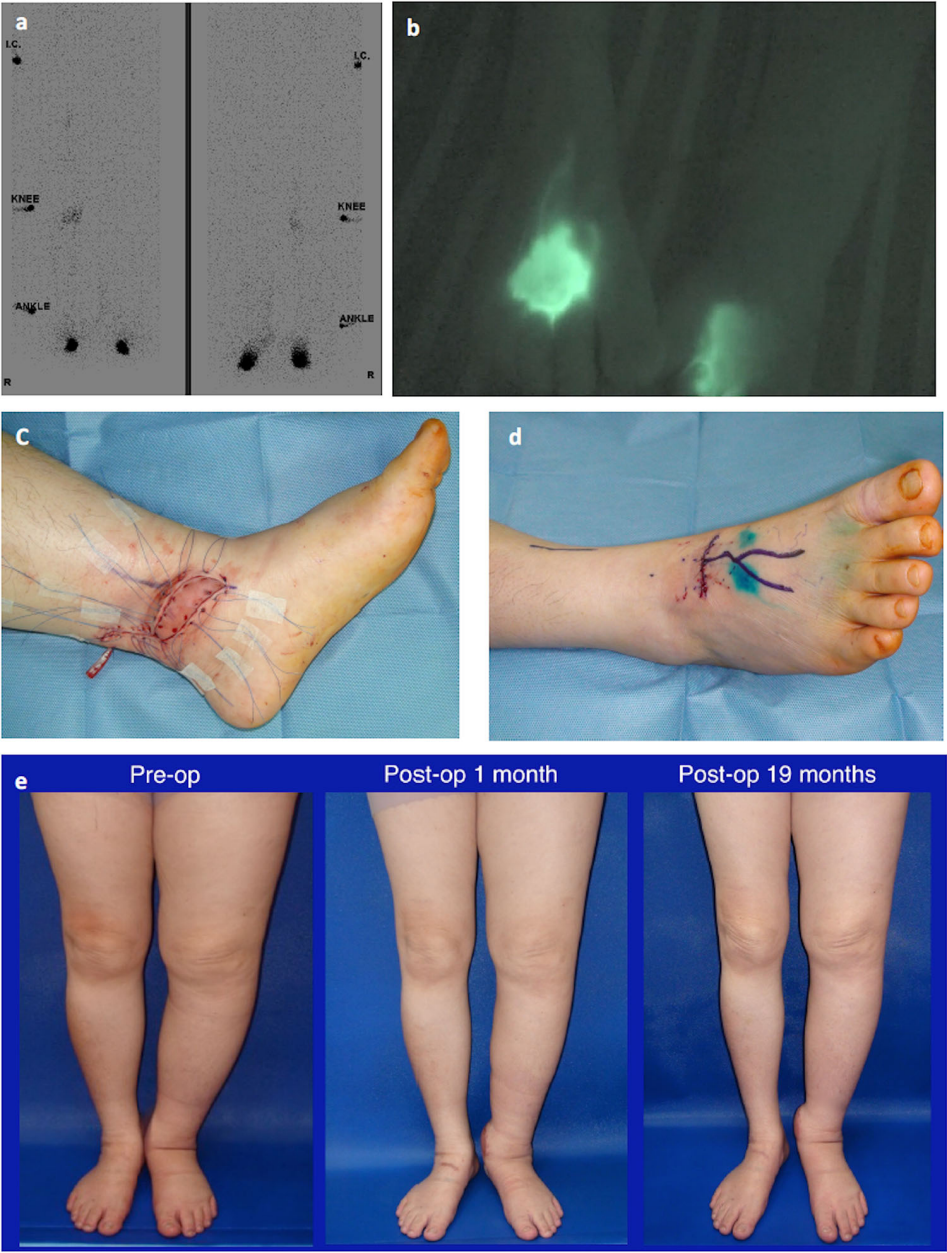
Diagnosis, surgical treatment, and outcome of a 68-year-old female (case 2) who suffered from bilateral lower extremity lymphedema of different severities. a Lymphoscintigraphy showed bilateral lymphedema, and the Taiwan Lymphoscintigraphy Staging results were more severe on the left (total obstruction) than right side (partial obstruction); b Indocyanine green lymphography showed patent lymphatic ducts in the right leg but diffuse dermal backflow in the left leg; c A right vascularized submental lymph node flap was harvested and transferred to left ankle with end-to-end anastomosis of the facial artery to the posterior tibial artery and end-to-end anastomosis of the facial vein to the great saphenous vein; d Two side-to-end lymphovenous anastomoses were performed on the right ankle; e Photographs taken preoperatively and at 19-month follow-up showed improvement in the right leg (at 4 and 3 cm above and below knee) and left leg (at 3 and 1.5 cm above and below knee). Her overall LYMQoL improved from 5 to 8 at 12-month follow-up
